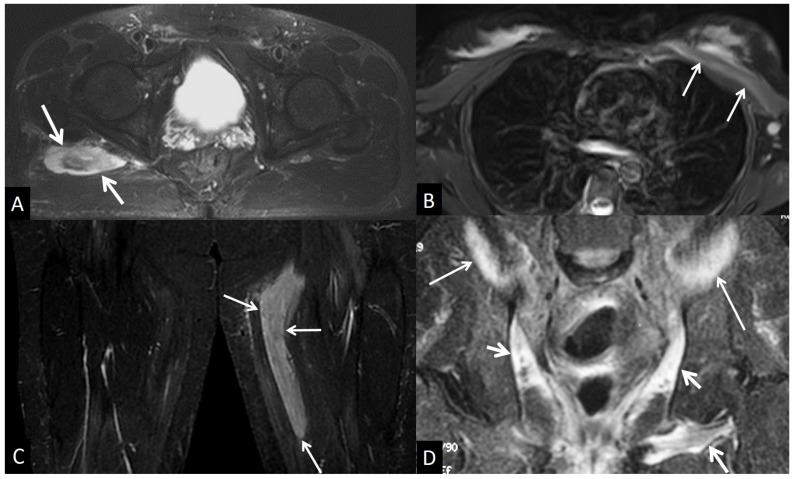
(A) Muscle contusion in a 65-year-old male following a fall from 2.5 m. Axial fat-suppressed T2-w MR image showing the intramuscular hematoma (arrows). (B) Axial fat-suppressed T2-w MR image showing the pectoralis major muscle strain (arrows). (C) Coronal STIR MR image showing muscular edema (arrows) in a 43-year-old female marathon runner with delayed muscle soreness syndrome. (D) Coronal STIR MR image in a 65-year-old male patient with bladder carcinoma, showing post-radiation changes in the iliac (thin arrows) and obturator (short arrows) muscles.
